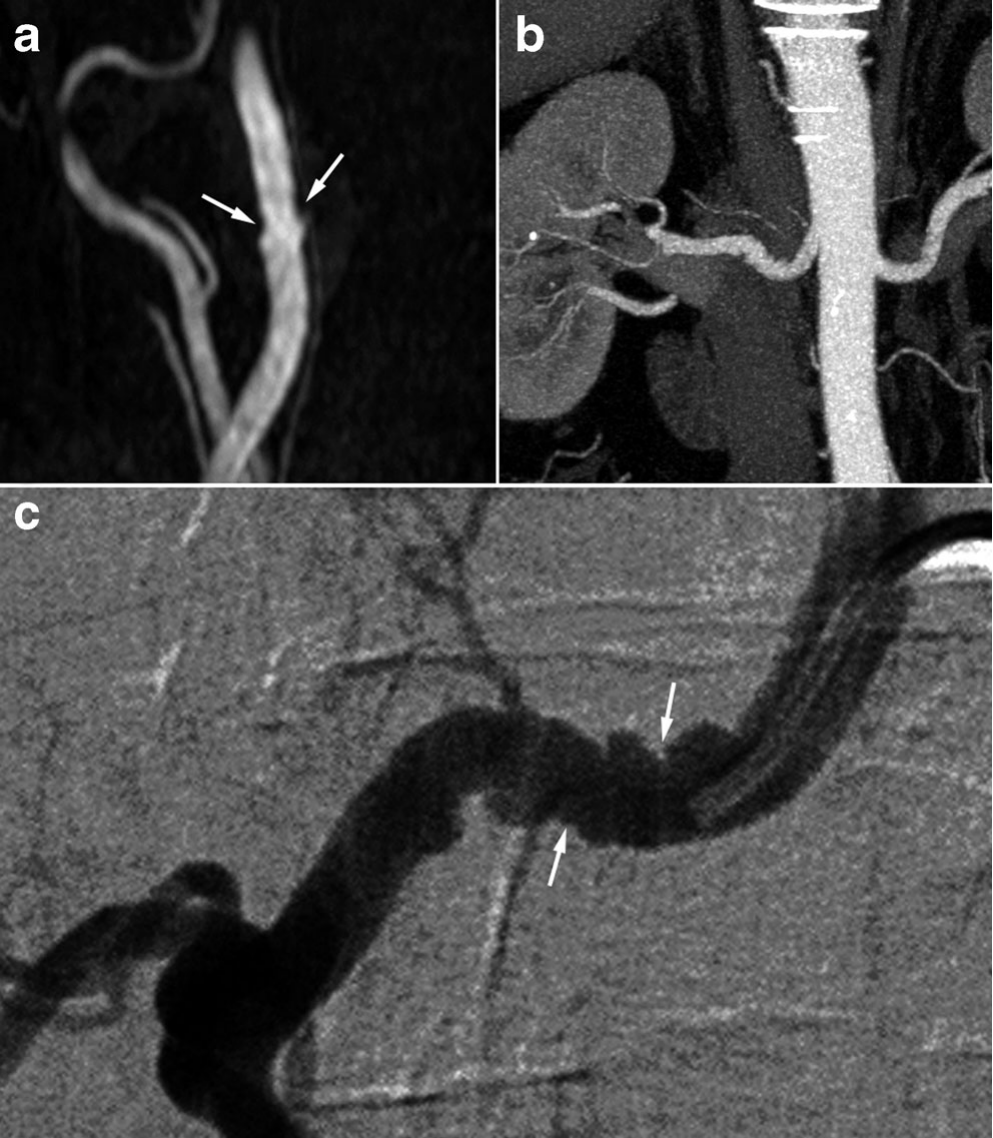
Radiologic findings in a 62-year-old male patient with arterial hypertension. a Gadolinium-enhanced MRA with MIP reconstruction showing typical but discrete involvement of the internal carotid artery (*white arrows*). b Renal CTA, coronal MIP reconstruction in the same patient showing a typical aspect of a medial string-of-beads dysplasia of the right renal artery. c Right renal angiogram confirming the typical aspect of the right renal artery, in favour of FMD
